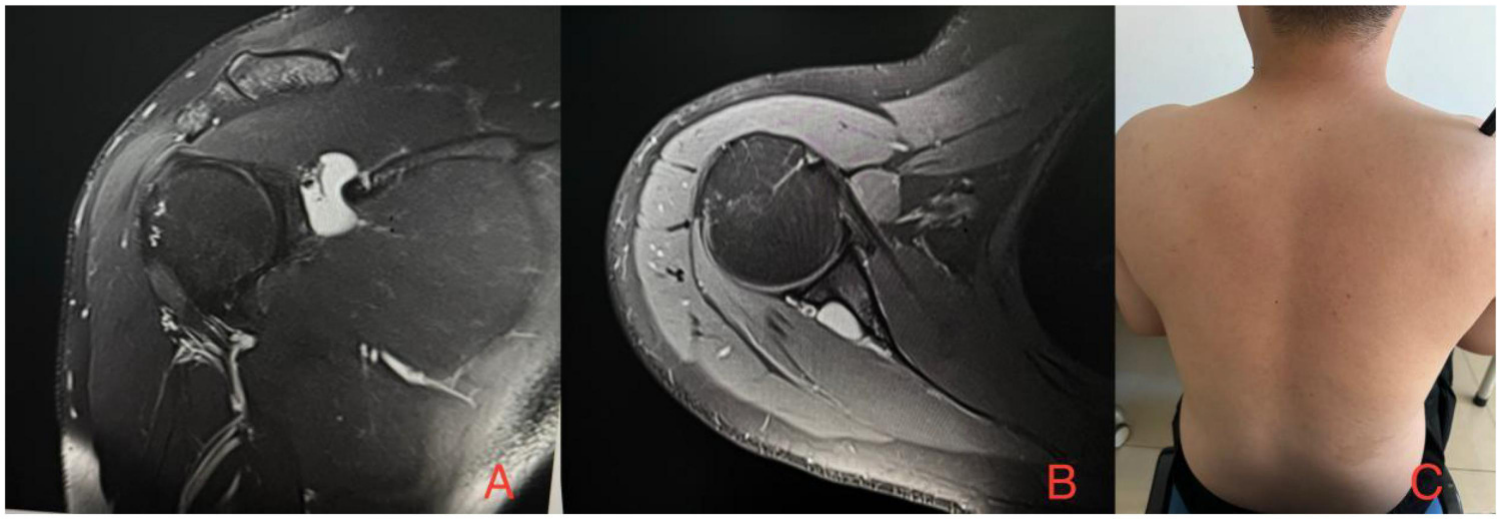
MRI of the right shoulder demonstrates a well-defined, unilocular abnormally hyperintense lesion (cyst) measuring approximately 2 cm × 2 cm at the spinoglenoid notch, which is in close proximity to the suprascapular nerve and adjacent vessels. (A), Coronal view. (B), Axial view. (C) Preoperative visual inspection revealed a mild depression in the right infraspinous fossa.
